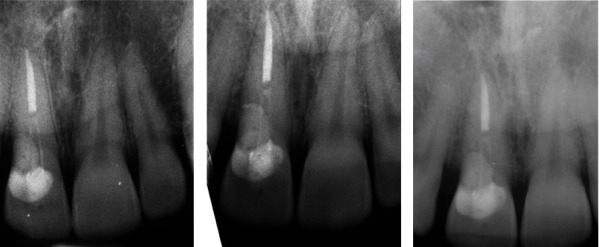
X-ray at (a) 1-year, (b) 2-year, and (c) 3-year follow-ups.
